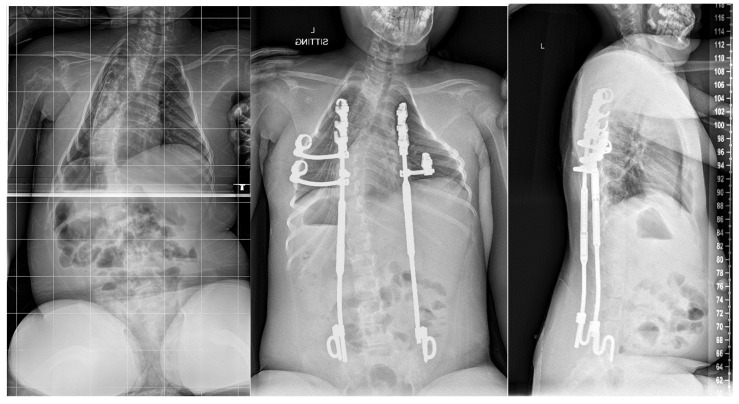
Pre- and postoperative radiographs of an 8-year-old male with Type Ib SMA showing insertion of modified MCGR construct with VEPTR anchors and rib gantries.
